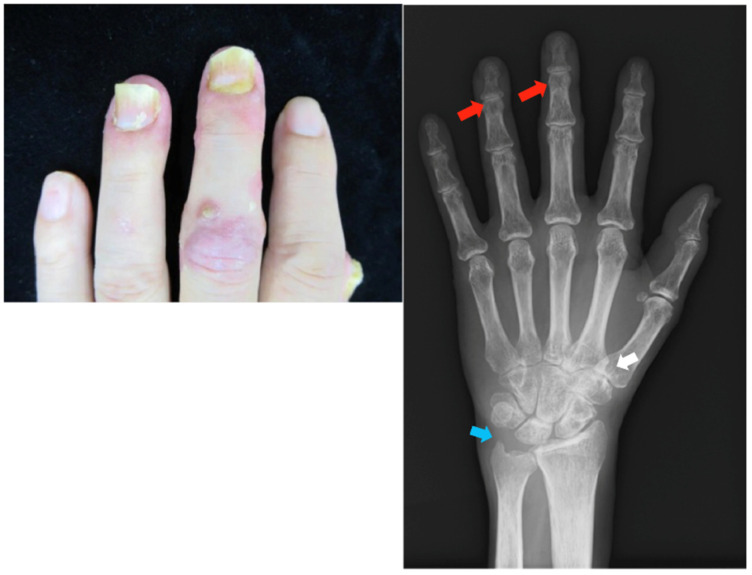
Clinical photograph and radiograph of the hands in case 2. There is nail psoriasis in the left third and fourth fingers and tenderness in the same DIP joints. Joint space narrowing is depicted in the same DIP joints (red arrow). Additionally, joint space narrowing is shown in the left carpal joint (white arrow), and the left styloid process is smaller than the right one (blue arrow). DIP, distal interphalangeal.
